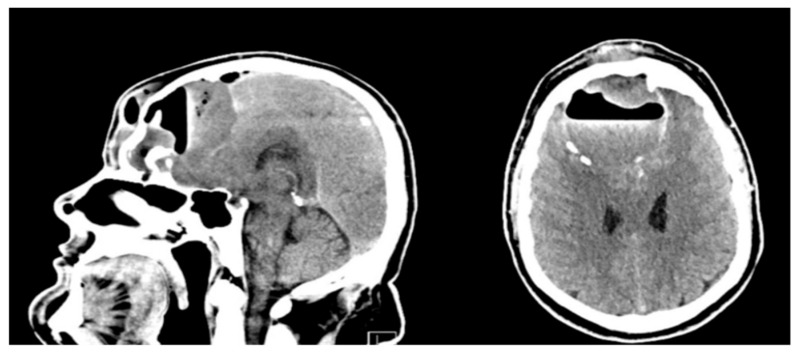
Head CT of the patient before surgery, showing a large structure with an air-fluid level, small gas bubbles, and peripheral calcifications bilaterally in the frontal lobes, brain tissue edema, a 4 mm midline shift, and subfalcine herniations, accompanied by destruction of the posterior frontal lobe. The imaging highly suggested PPT.
